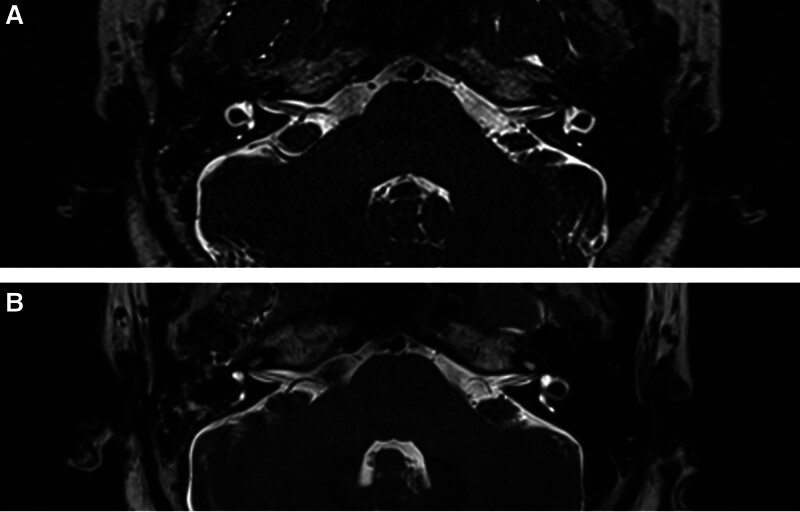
Magnetic resonance imaging (T2W_TSE) of the inner ear. (A) Preoperative. (B) Two weeks after surgery. T2W_TSE = T2-weighted turbo spin echo.
